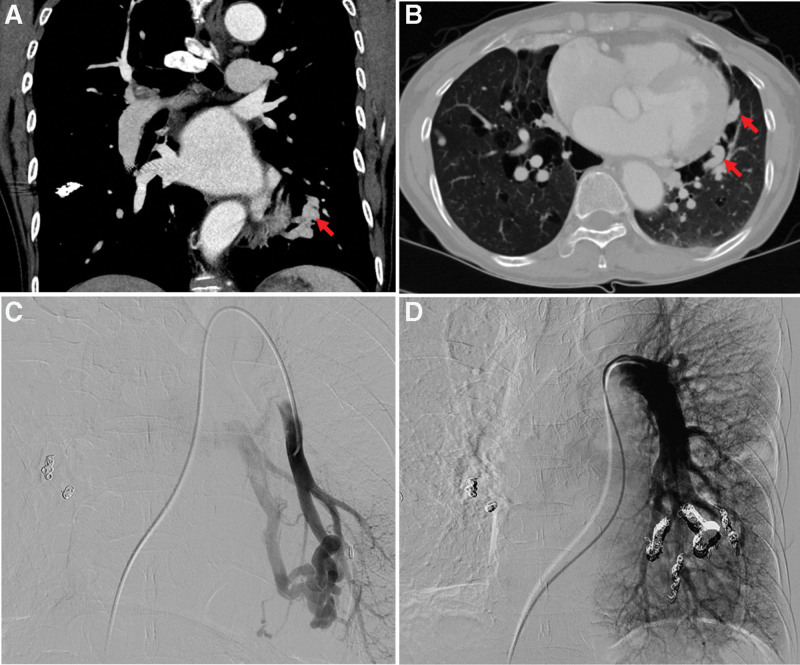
The follow-up chest CT images and angiographic findings 11 years after the first embolization. (A and B) Follow-up chest CT image 11 years after the first embolization demonstrates newly appeared multiple AVMs in the LLL of the lung, which were not seen in the initial chest CT scan. (C) The selective angiography of the left basal segmental pulmonary artery confirms the AVM in the LLL. After coiling of this branch, the posterior basal and inferior lingular AVMs were sequentially embolized with microcoils. (D) The final angiography demonstrates complete occlusion of the AVMs. AVMs = arteriovenous malformations, CT = computed tomography, LLL = left lower lobe.
